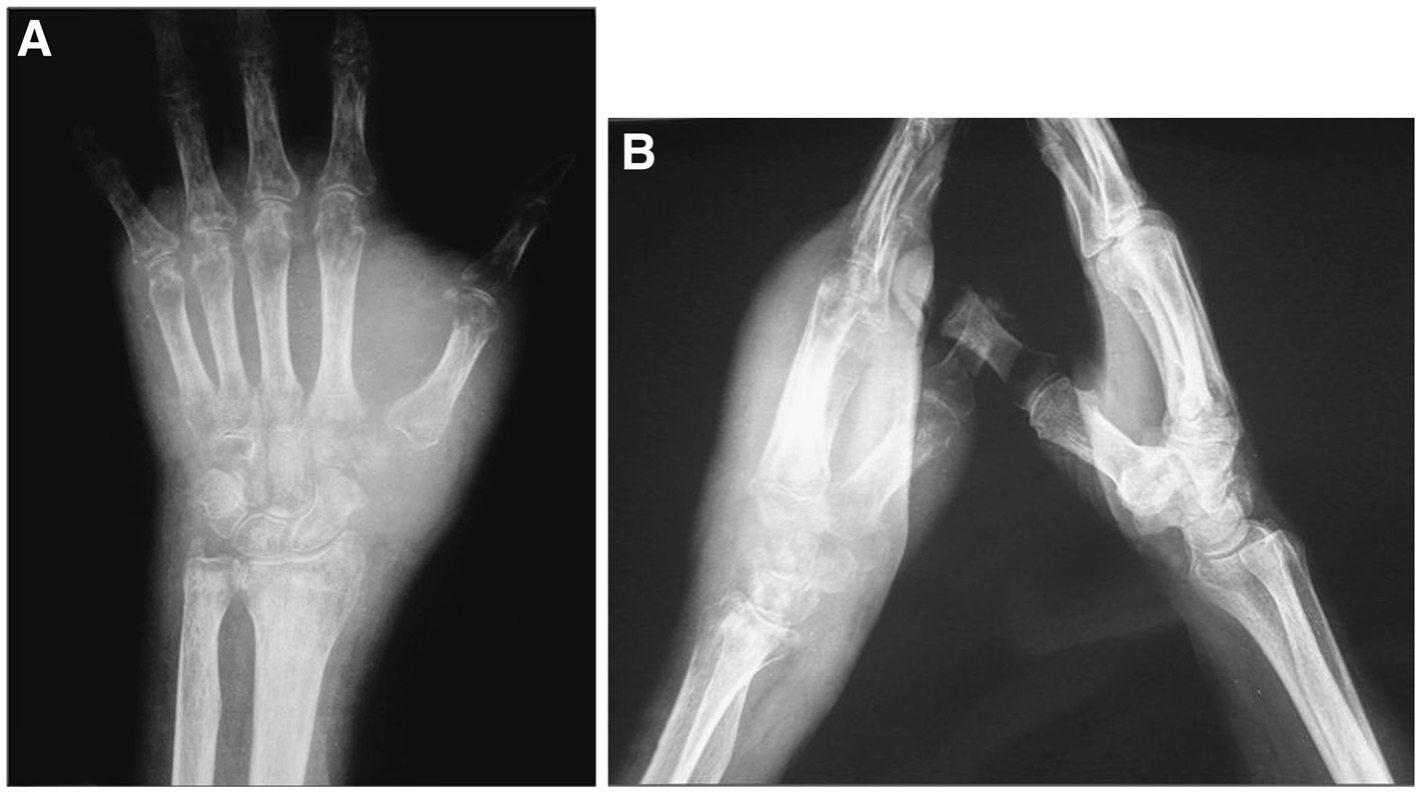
Plain radiograph of the left hand: almost total resorption of the trapezium and trapezoid. Diffuse osteoporosis. (A) Anteroposterior view. (B) Lateral view of both hands.
